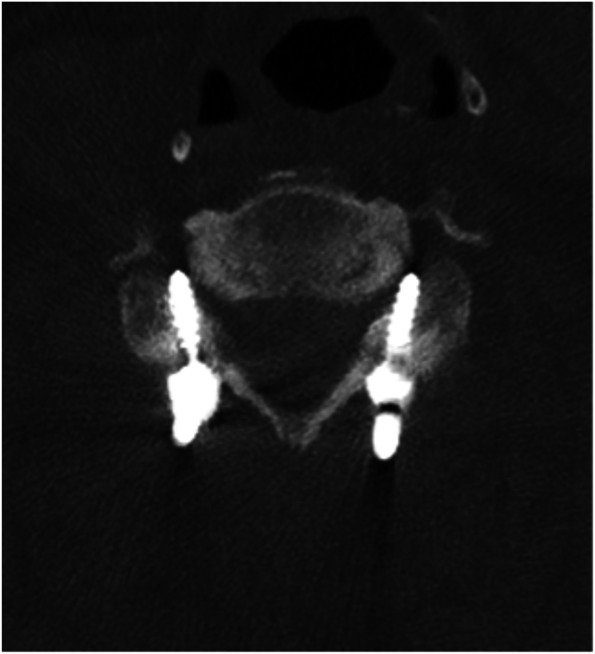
An axial computed tomography slice of the same patient at C6 level. Because of increasing nerve root symptoms, a computed tomography was taken. This slice shows lateral mass screws penetrating the nerve root channels bilaterally.
